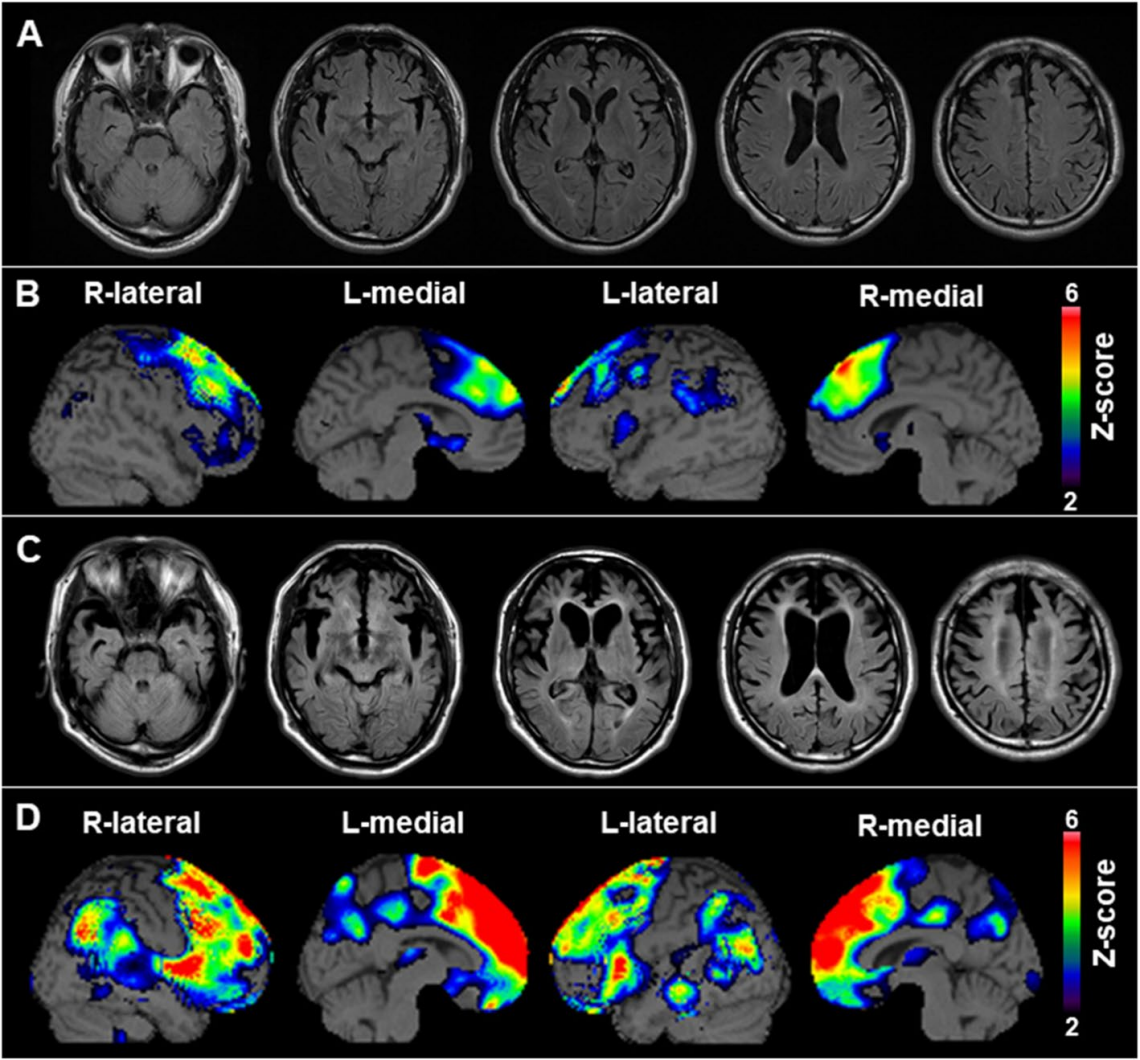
Neuroradiological findings. a Transverse fluid-attenuated inversion recovery (FLAIR) magnetic resonance imaging (MRI) shows bilateral frontal lobe atrophy. b Brain perfusion single-photon emission computed tomography (SPECT) easy Z-score Imaging System (eZIS) analysis (FUJIFILM Toyama Chemical Co., Ltd., Tokyo, Japan) shows relative hypoperfusion in the frontal lobe. The color scale for the Z score is shown in the right part of the figure. The colored areas represent Z scores > 2. c Transverse FLAIR MRI performed 10 years later shows remarkable bilateral frontal and temporal lobe atrophy. d eZIS analysis performed 10 years later reveals remarkable hypoperfusion in the frontal and parietal lobes
